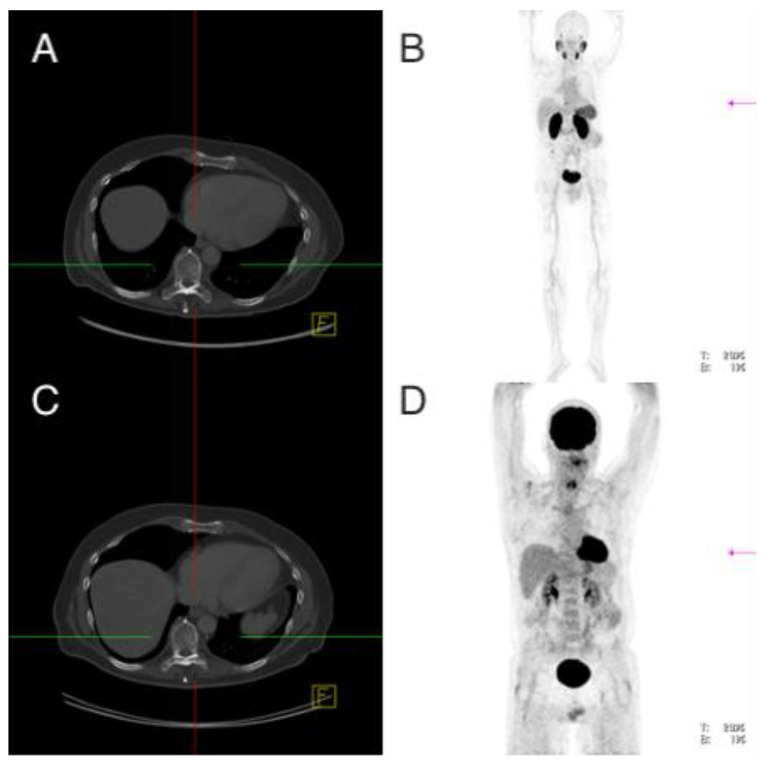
Patient 8. ^68^Ga-PSMA-PET/CT (A,B), ^18^FDG-PET/CT (C,D). Morphologic pattern of thoracic vertebral body 9 with osseous metastasis in CT.
